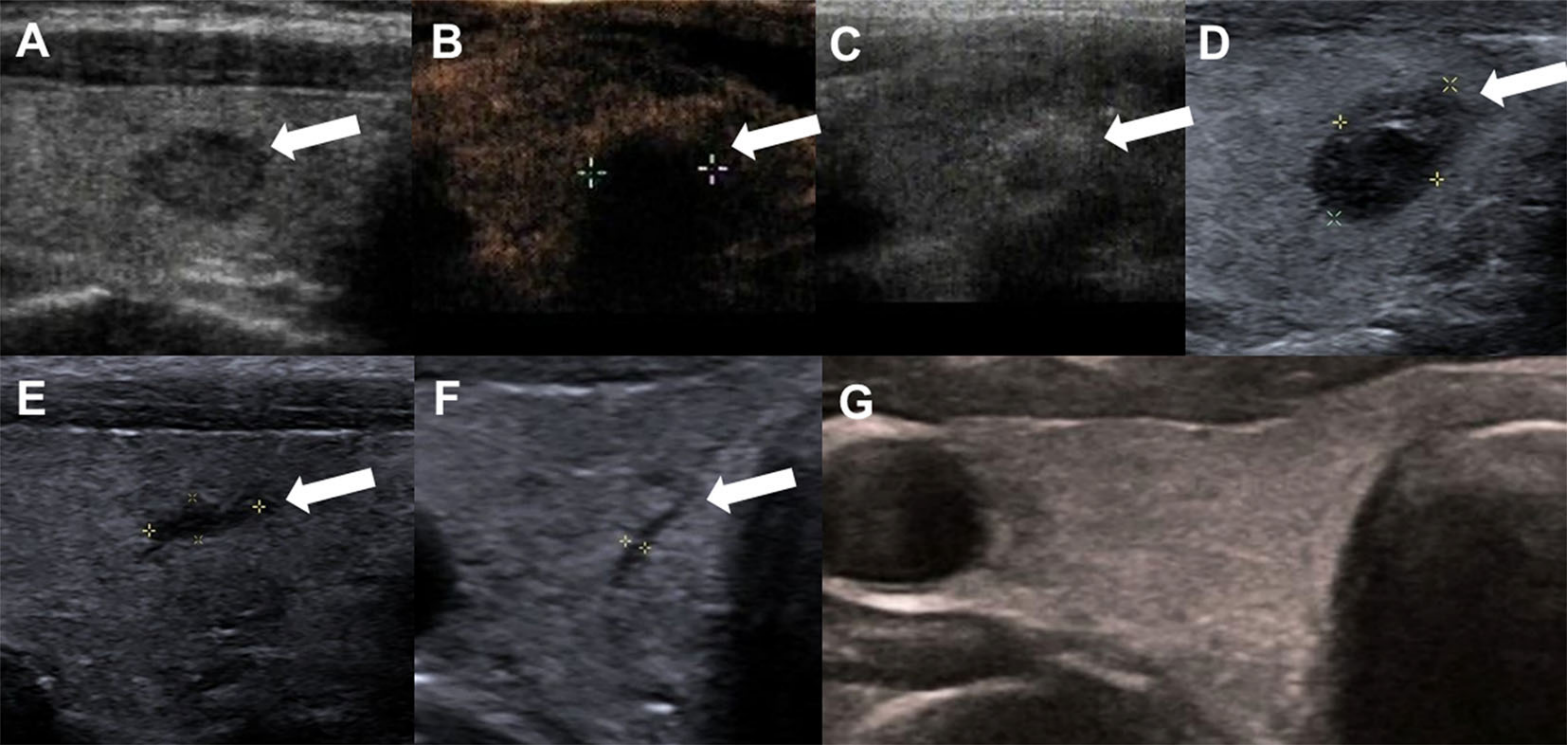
A 47-year-old woman with Bethesda IV thyroid nodules on FNA in the right thyroid lobe. (A) Before RFA, ultrasound image shows a hypoechoic nodule with an smooth margin thyroid nodule, and size with 0.9cm×0.6 cm×0.6 cm; (B) Contrast-enhanced ultrasound performed immediately after RFA shows larger in size(1.2cm×1.1cm×1.1 cm) than that before ablation and a complete lack of enhancement in the treated area (white arrows). (C) Ultrasound shows gas formation in the nodule which covered by a hyperechoic ablation area (white arrows); (D) 1 month after ablation, the ablation zone was 0.9cm × 0.5cm × 0.6 cm in size; (E) 3 month after RFA, ultrasound shows the treated area shrunk (0.6cm×0.2cm×0.2cm); (F) 6 months after RFA, ultrasound shows line-like hypoechoic area, remained as a small scar lesion (white arrows); (G) The ablation area disappeared on ultrasonography 12 months after ablation.
